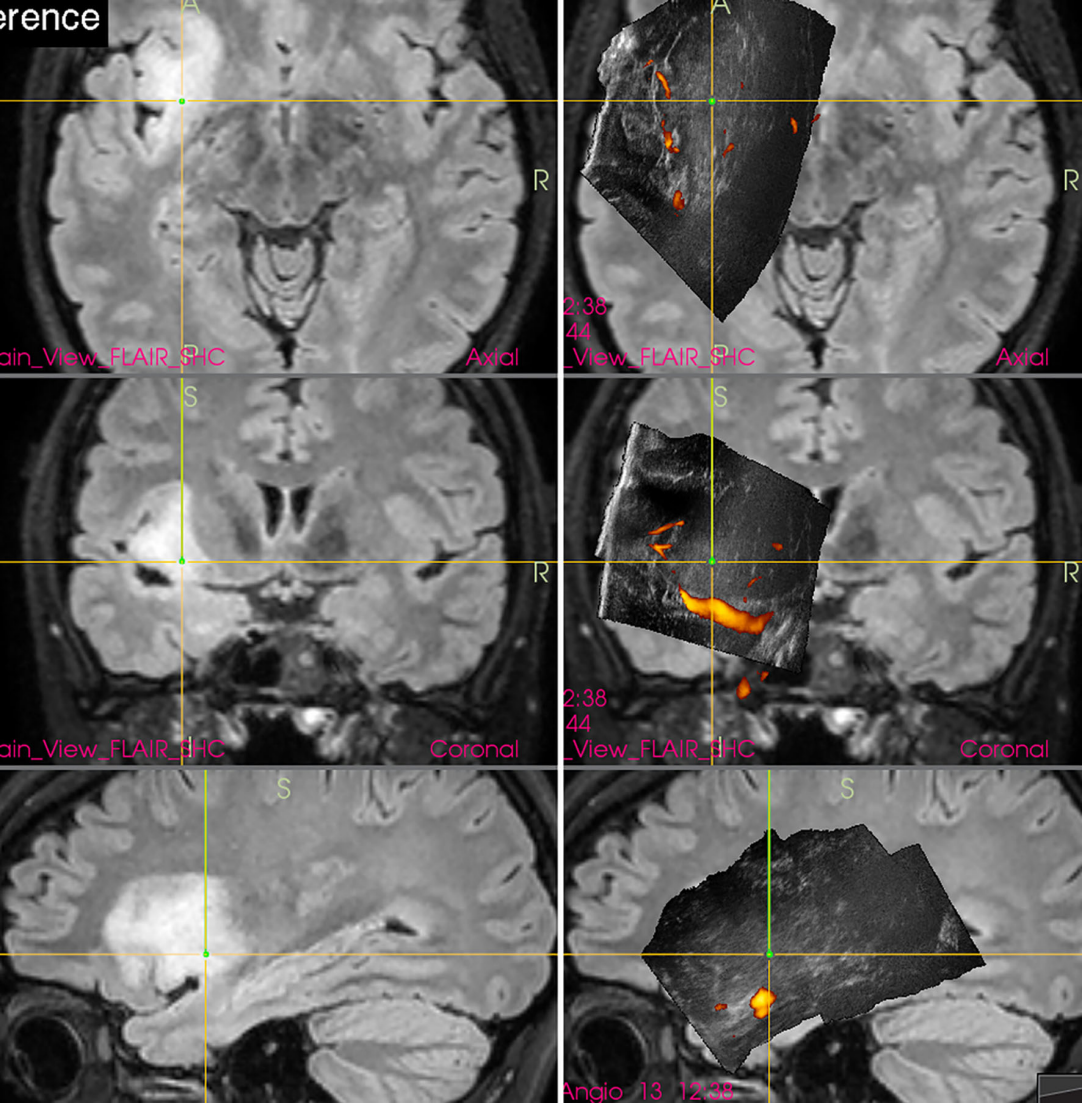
Left-sided insular grade II astrocytoma (Left column) preoperative navigation 3D FLAIR MRI sequence (3-Tesla MRI scanner) (Right column) pre-resectional 3D IOUS image fused with navigation FLAIR MRI sequence. Note that the tumor tissue is only mildly hyperechoic and less distinctly visualized comparing to MRI. 3D, three-dimensional; IOUS, intraoperative ultrasound; FLAIR, fluid attenuated inversion recovery; MRI, magnetic resonance imaging.
